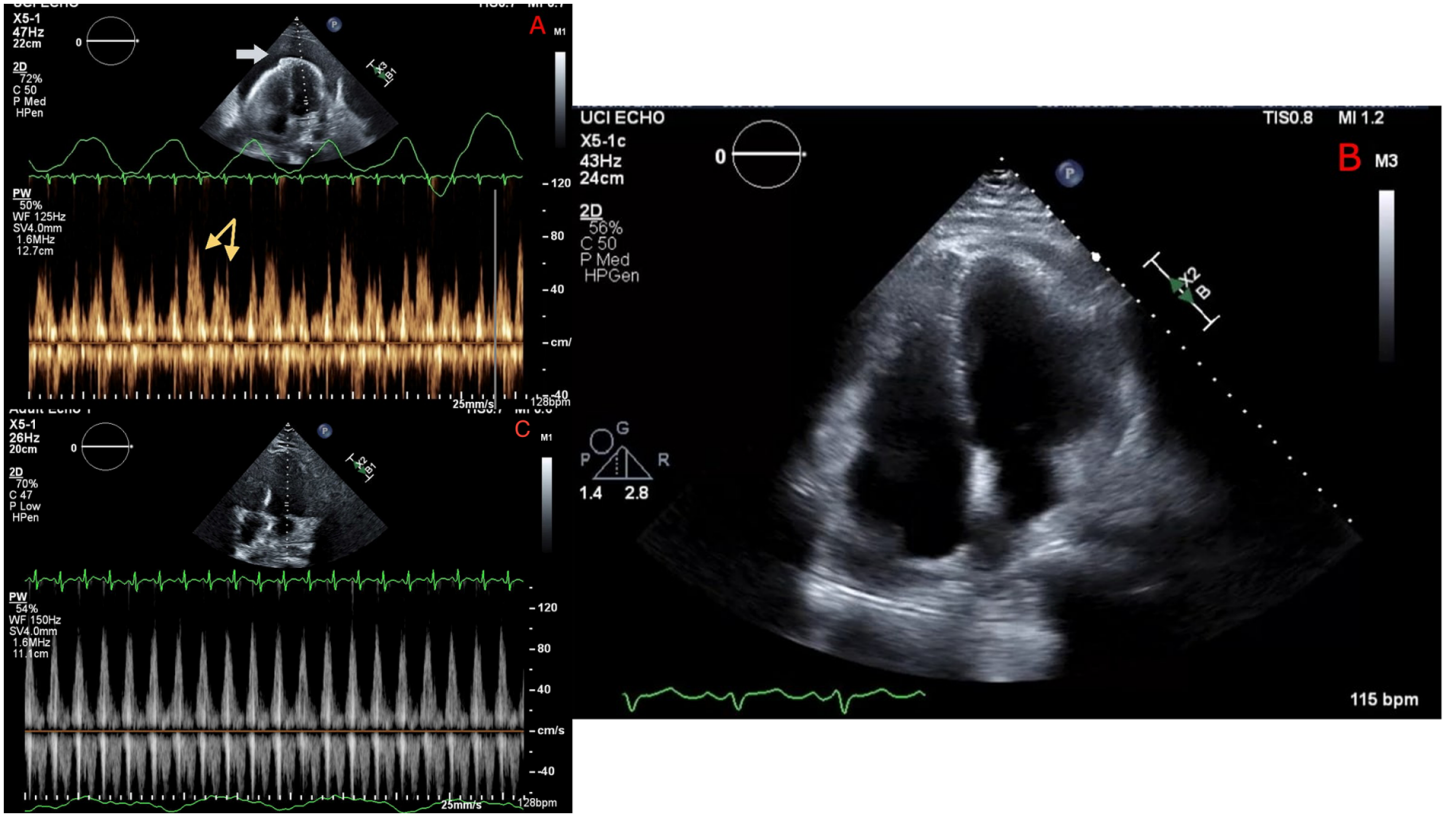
Echocardiography before pericardiocentesis (A) showing a large pericardial effusion (white arrow), with Doppler revealing significant respiratory variation of mitral inflow velocity (yellow arrows) consistent with tamponade. Repeat echocardiography immediately following pericardiocentesis (B) showing resolution of pericardial effusion and (C) no significant respiratory mitral valve inflow variation.
